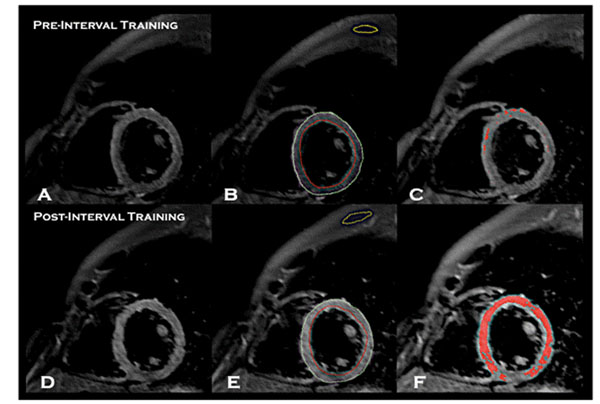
Visibly increased extent of myocardial edema in an endurance athlete following high-intensity interval training using T2-weighted cardiac MRI imaging (panel D vs. panel A). Extent of myocardial edema was quantified by tracing endocardial (red contours) and epicardial contors (green contours) to determine myocardial signal intensity, as well as yellow contours to measure skeletal muscle signal intensity (panels B, E). Mean signal intensity of skeletal muscle was multiplied by a factor of 2, and this number was utilized as a threshold to semi-automatically detect the extent of myocardial edema (red overlay, panels C and F). In this example, the extent of myocardial edema increased from 1.5% to 59.6% of LV mass following intense interval training.
